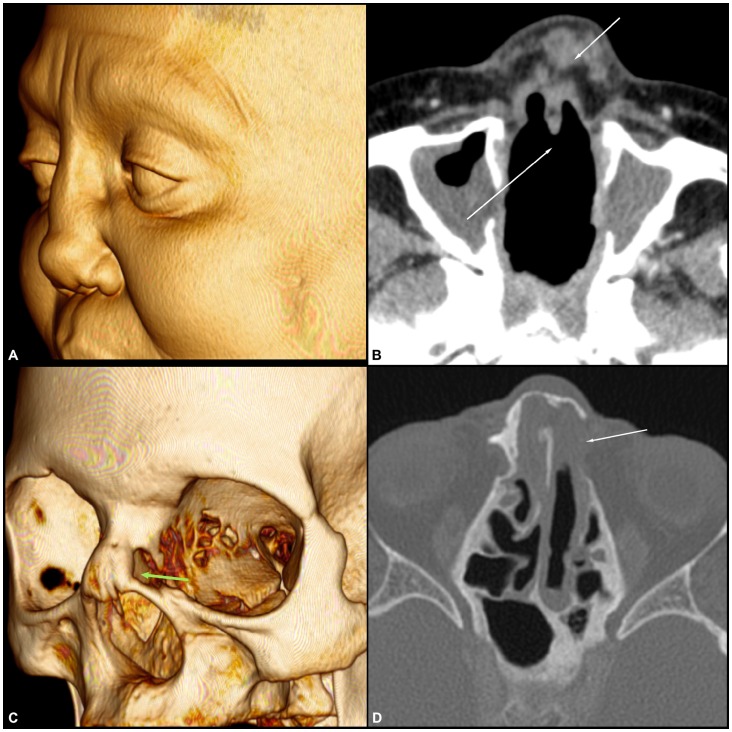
Volume-rendered 3D images of multi-slice CT data showing diffuse thickening of the nasal wings with collapse of the nasal pyramid (A) and erosion of the nasal bone (C). Axial computed tomography (CT) scan with soft tissue window (B) showing diffuse thickening of the nasal wings with collapse of the nasal pyramid, erosion of the nasal septum and opacification of the maxillary sinuses. Axial computed tomography (CT) scan of the bone window (D) demonstrates the erosion of the nasal bone and partial opacification of the ethmoid air cells.
